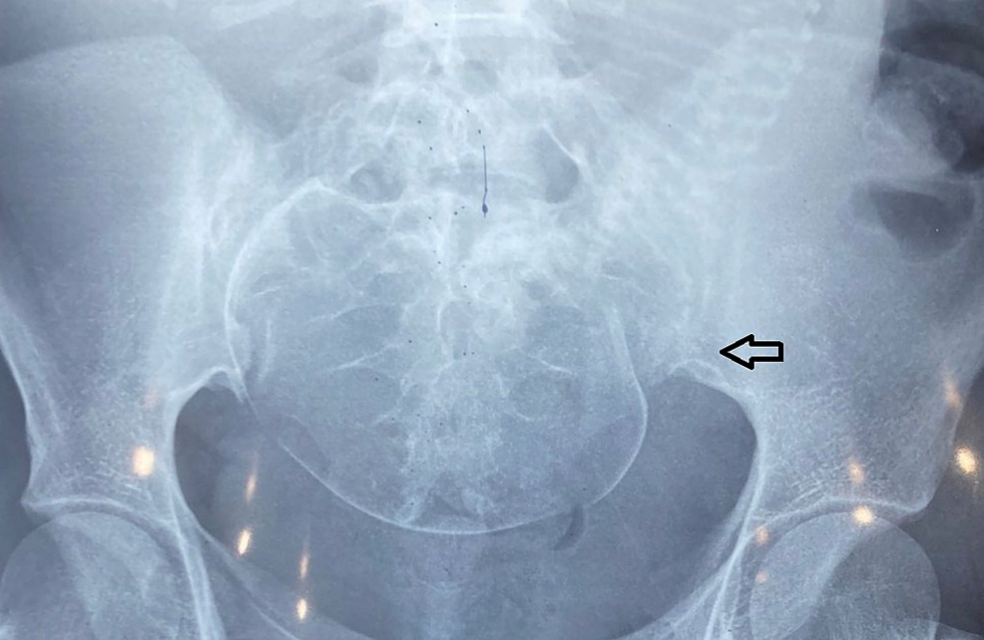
X-ray bilateral sacroiliac view showing left-sided sacroiliitis with fetus in-situ The arrow shows left-sided sacroiliac joints (affected side) contrasted with normal right-sided sacroiliac joint.
